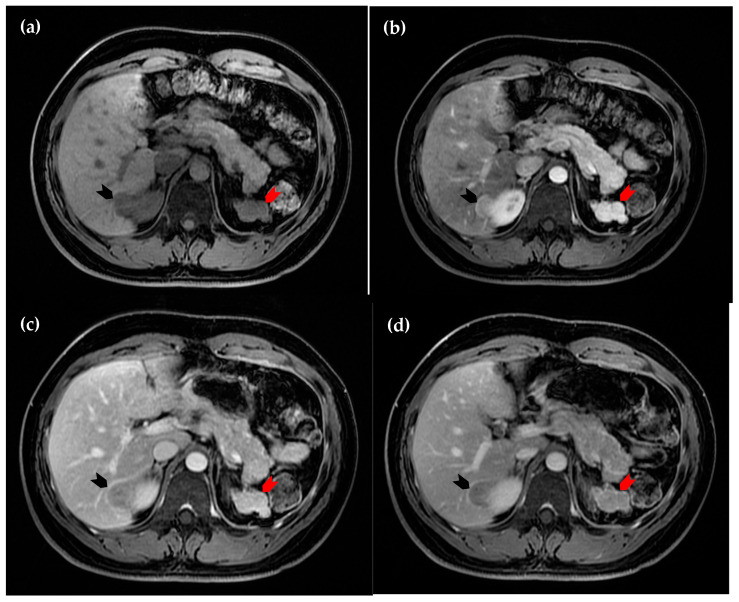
Dynamic magnetic resonance imaging of the liver. (a) Axial view of the pre-contrast sequence, in which the intrahepatic lesion is hypointense (black arrowhead); (b) axial view of the arterial phase, in which the lesion demonstrates homogeneous hyperintensity (black arrowhead); (c) axial view of the portal phase, in which early wash-out of enhancement is observed (black arrowhead); (d) axial view of the delayed phase, in which the lesion (black arrowhead) remains more hypointense compared to the surrounding hepatic parenchyma. The perinephric lesion again demonstrates similar attenuation across all sequences (red arrowhead in (a–d)). However, due to the suspicious enhancement pattern of the intrahepatic lesion, a hepatic tumor cannot be excluded [1,2,3,4].
